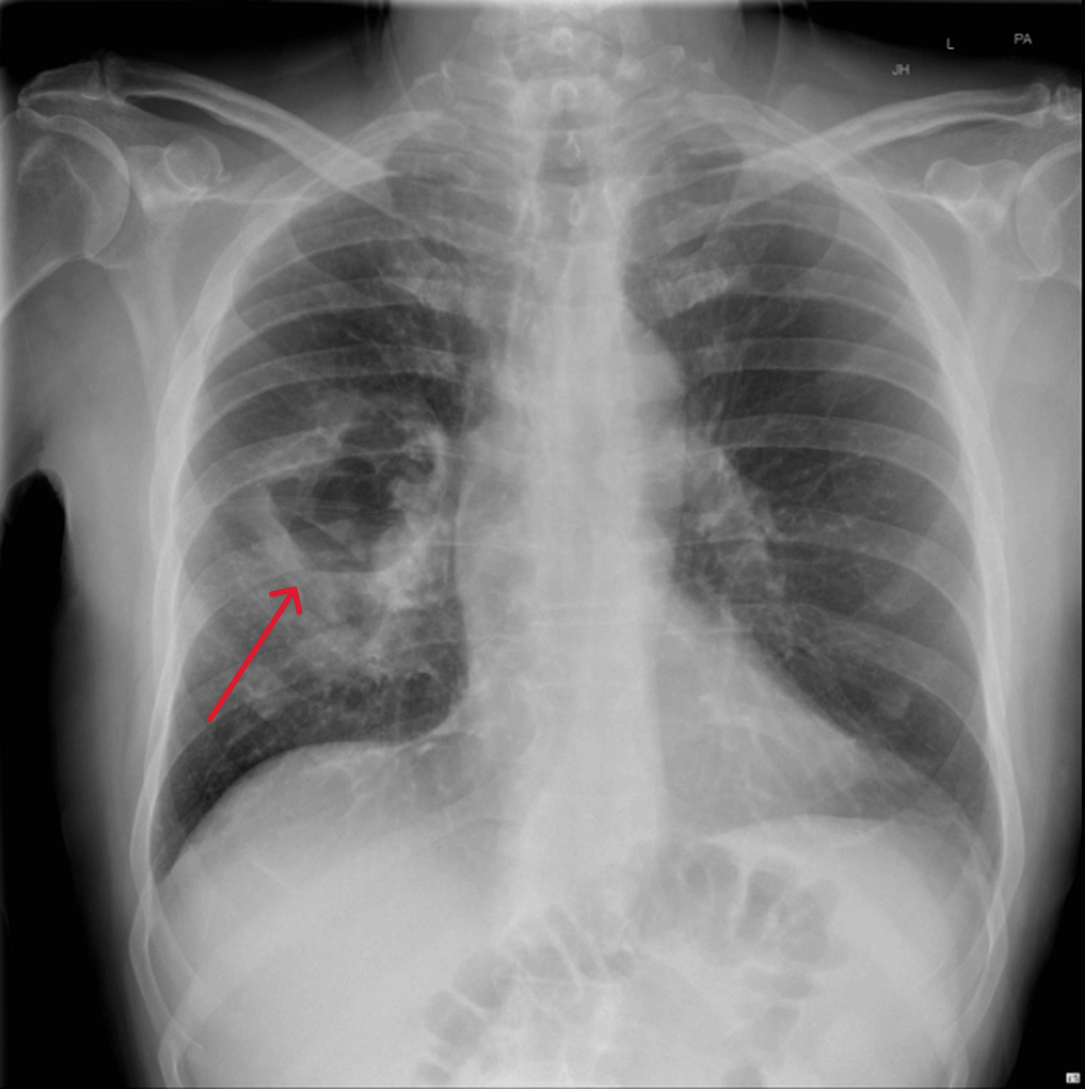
Chest x-ray (CXR) on admission The red arrow points toward the cavitating lesion seen in the right mid-zone.
